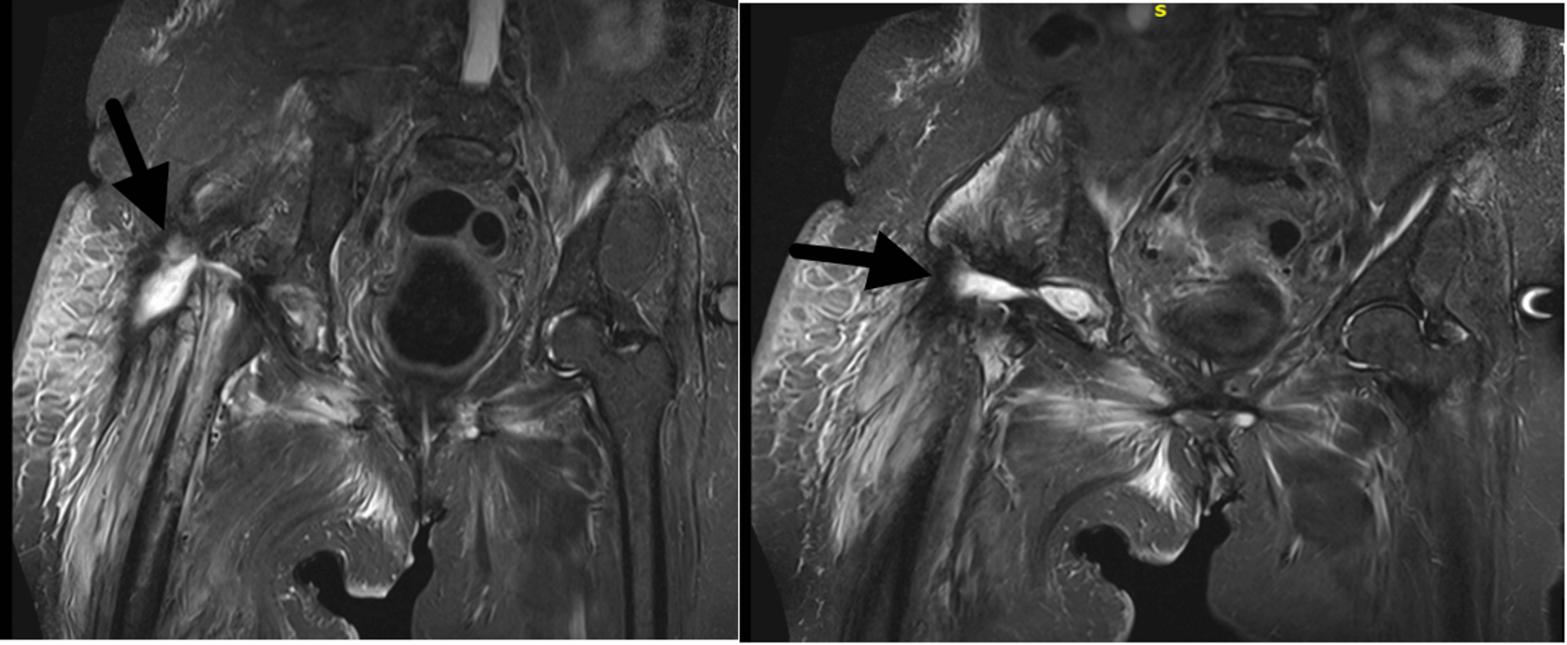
MRI demonstrating further improvement MRI: magnetic resonance imaging
